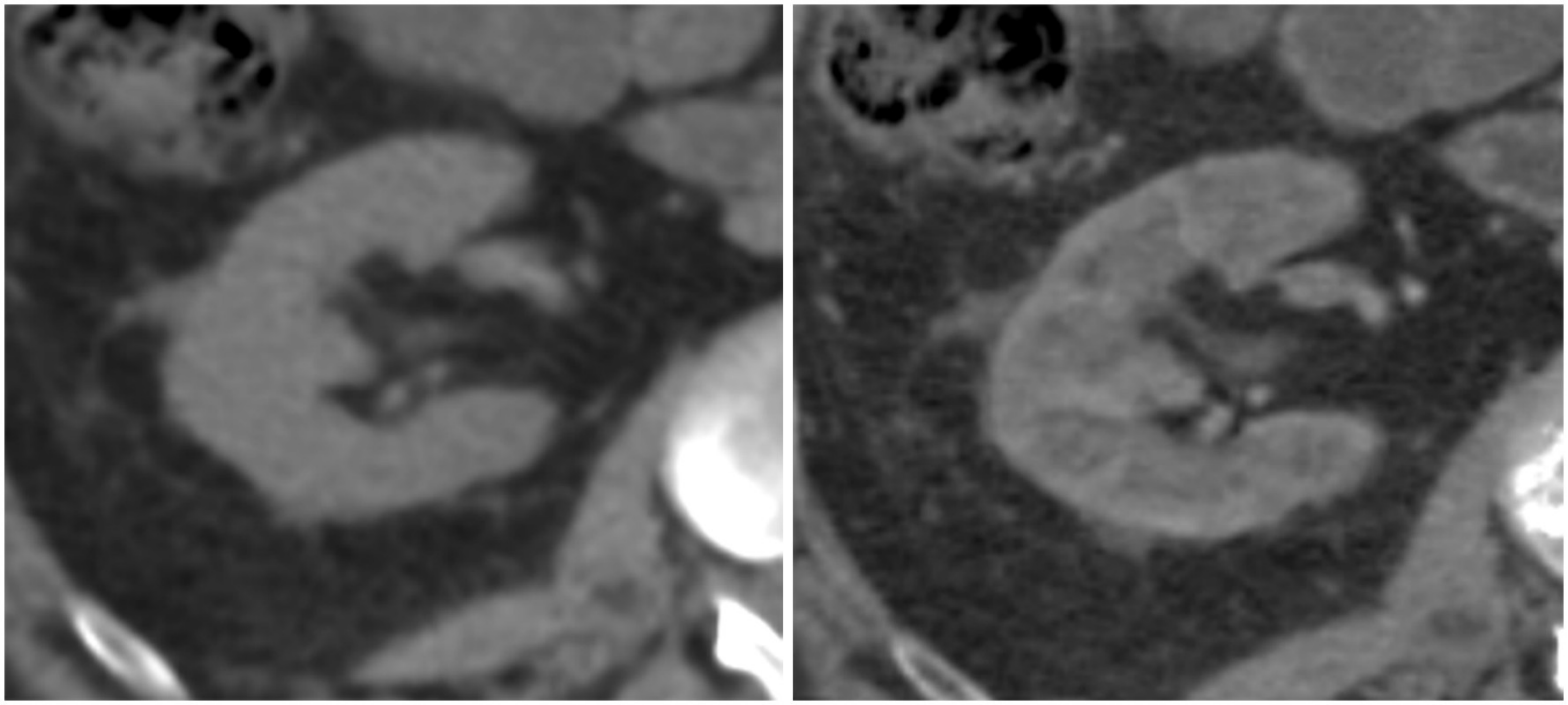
No cortico-medullary differentiation is visible on true unenhanced (TUE) images (left) while virtual unenhanced (VUE) images demonstrate cortico-medullary differentiation (right). Images are displayed using the same CT window width and window level.
